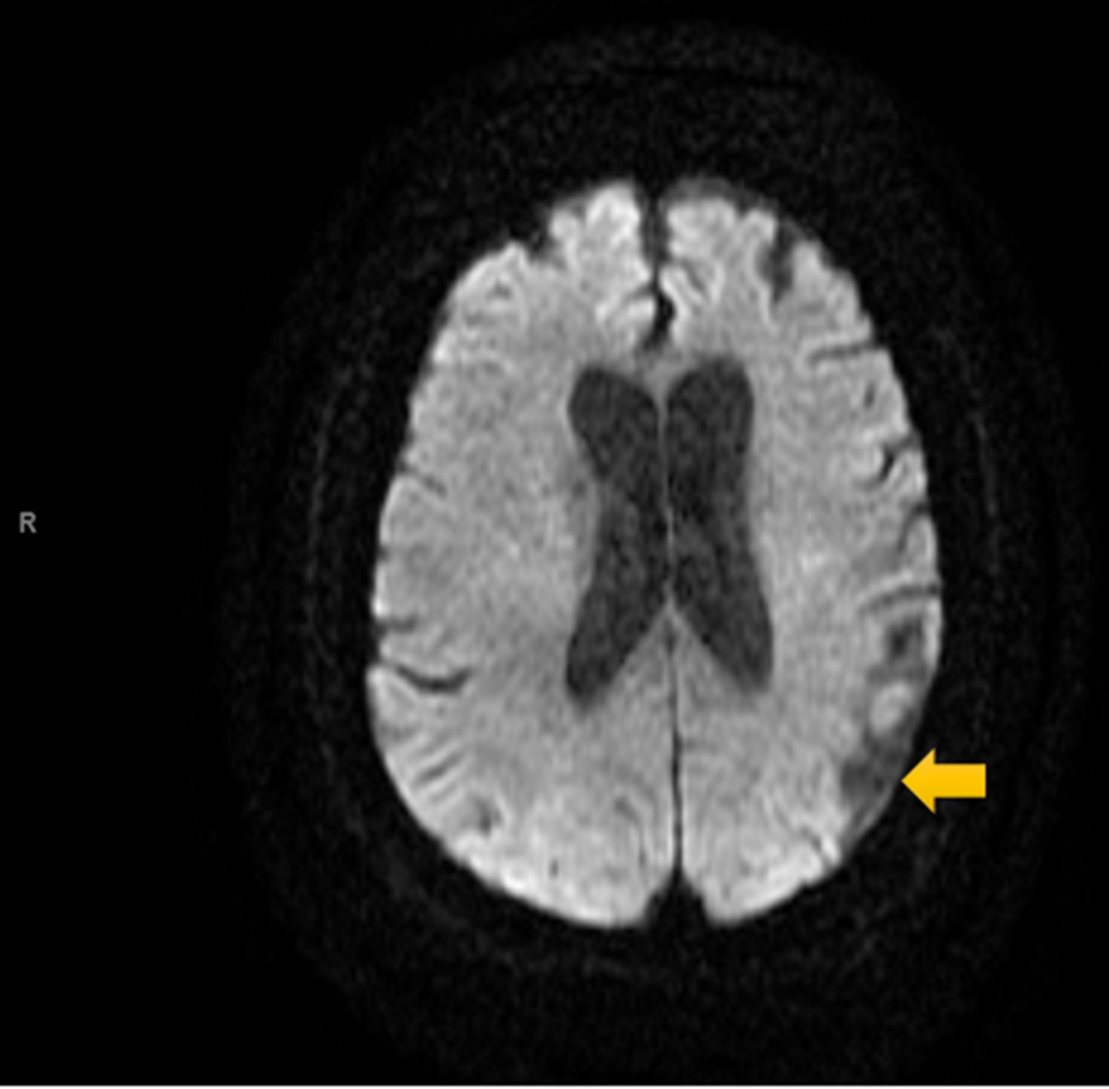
Diffusion-weighted MRI Scan of the Head Non-contrast diffusion-weighted MRI showing an old infarct in left parietal-temporal-occipital lobe.
